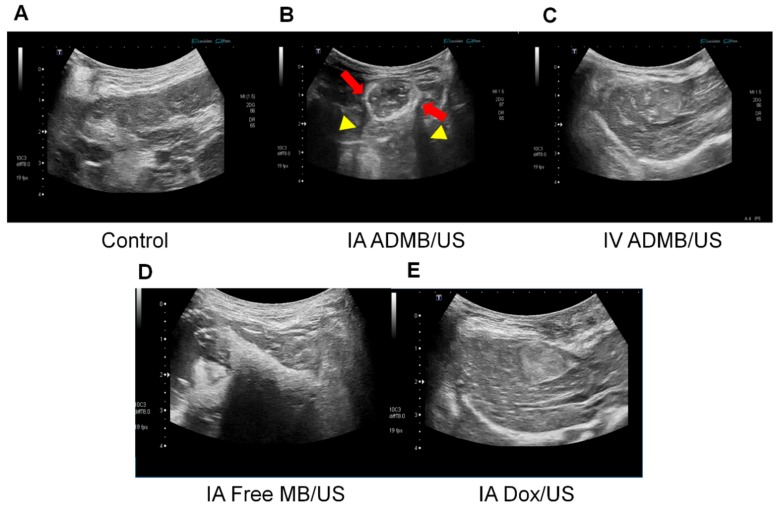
Representative ultrasound images of (A) control, (B) intraarterial injection of ADMB (IAMB/US), (C) intravenous injection of ADMB (IVMB/US), (D) intraarterial injection of microbubble (IA Free MB/US) and (E) intraarterial doxorubicin (IA Dox/US). Note that strong rim-like enhancement of intratumoral vessels (arrow) with posterior shadow (arrowhead) in IAMB/US group.
